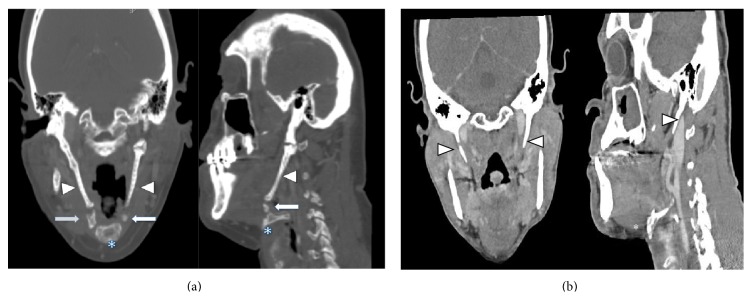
(a) CT scan of hyoid bone-styloid process extension. CT scan of hyoid bone and styloid process in patient. Arrowhead points to styloid process. Asterisk marks hyoid bone body. Thick arrow points to area of extension between hyoid bone and styloid process. (b) Normal CT scan of styloid process and hyoid bone. Normal CT scan of hyoid bone and styloid process for comparison. Arrowhead points to styloid process. Asterisk marks hyoid bone body.
